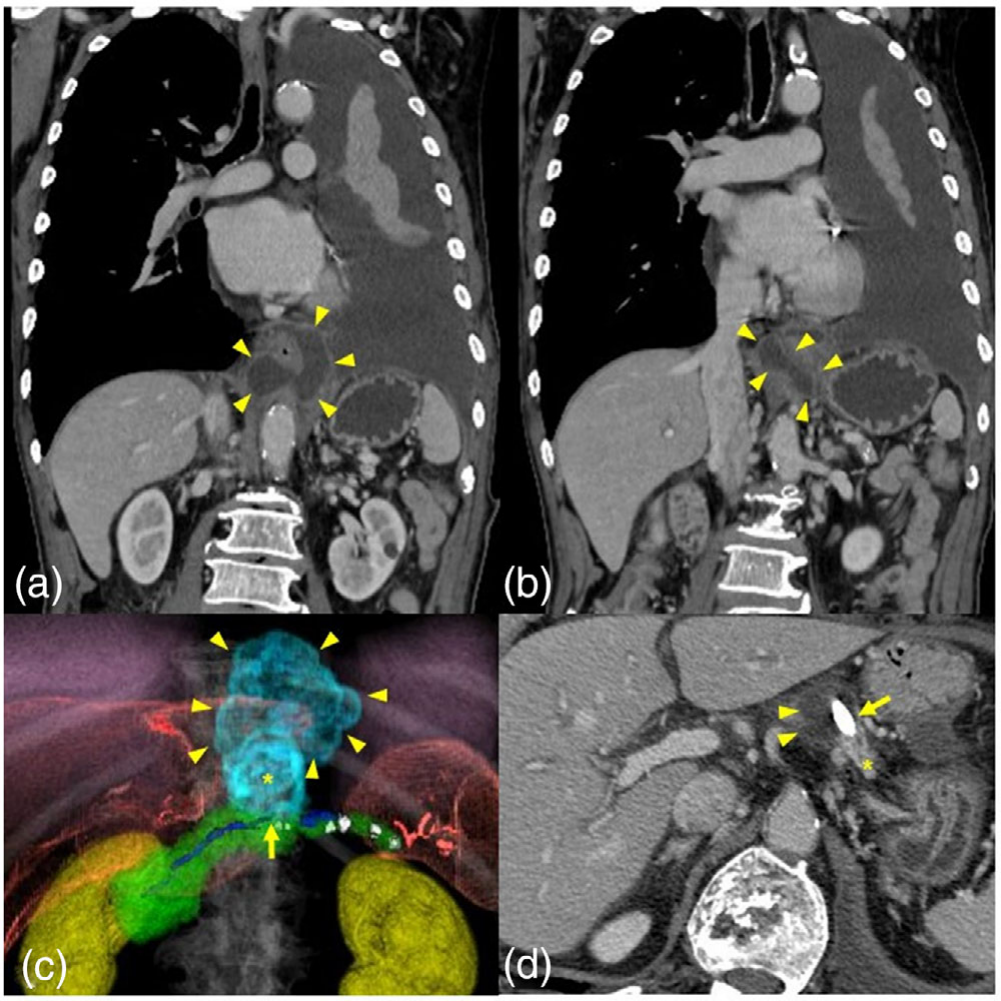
Chest and abdominal computed tomography: (a, b) A pancreatic pseudocyst extended toward the mediastinum and communicated with a mediastinal pseudocyst through the aortic hiatus (yellow arrowhead: pancreatic and mediastinal pseudocyst). (c) Three‐dimensional reconstructed computed tomography showed a connection between the pancreatic pseudocyst and mediastinal pseudocyst (green structure: pancreas, blue line: pancreatic duct, white structure: pancreatic stone, yellow arrowhead: mediastinal pseudocyst, asterisk: pancreatic pseudocyst, yellow arrow: pancreatic duct disruption). (d) The main pancreatic duct had ruptured toward the posterior surface of the pancreas on the proximal side of the pancreatic stone (yellow arrowhead: pancreatic duct rupture, yellow arrow: pancreatic stone, asterisk: pancreas)
